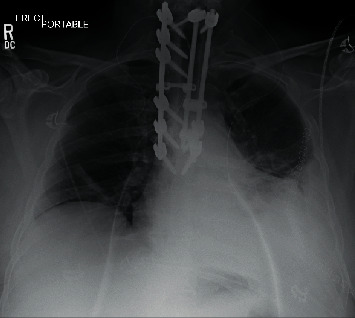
Chest X-ray of patient with COVID-19 pneumonia.
